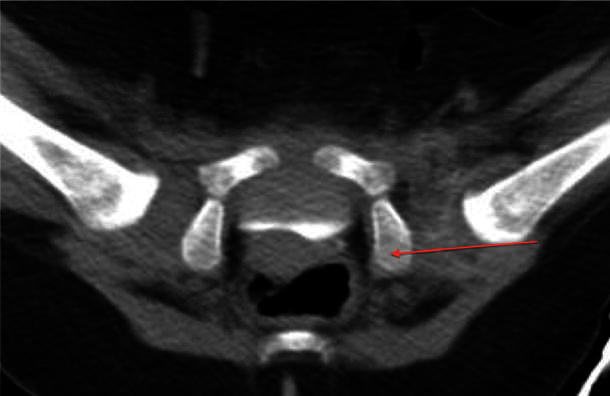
Postoperative single slice CT image with the posterior neck line revealing a dislocated left hip
